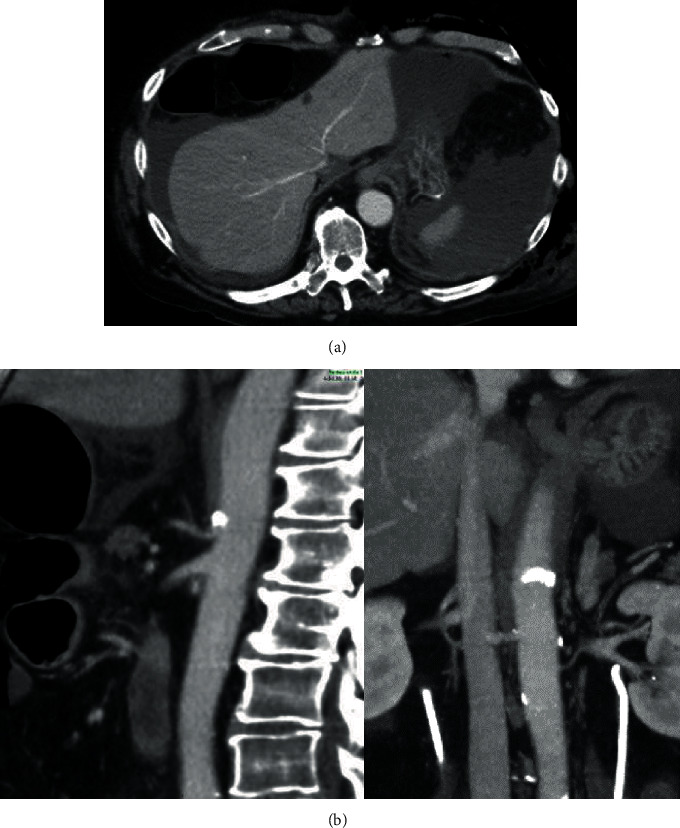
(a) CT with portovenous contrast shows intra-abdominal fluid around the stomach, spleen, and liver with free air suggestive of perforation (case 2). (b) CT lateral and axial views show a small-caliber coeliac trunk with calcification at the origin (case 2).
